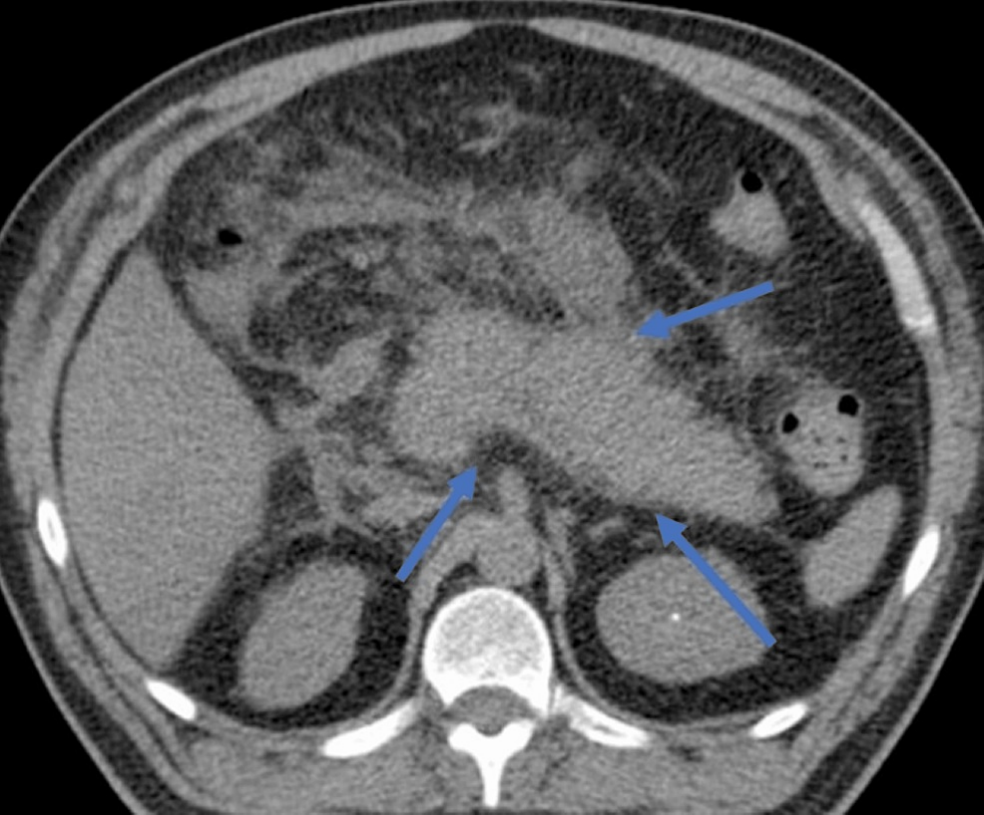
Abdominal computed tomography revealing diffuse pancreatic enhancement with fat stranding and peripancreatic fluid (blue arrows).
